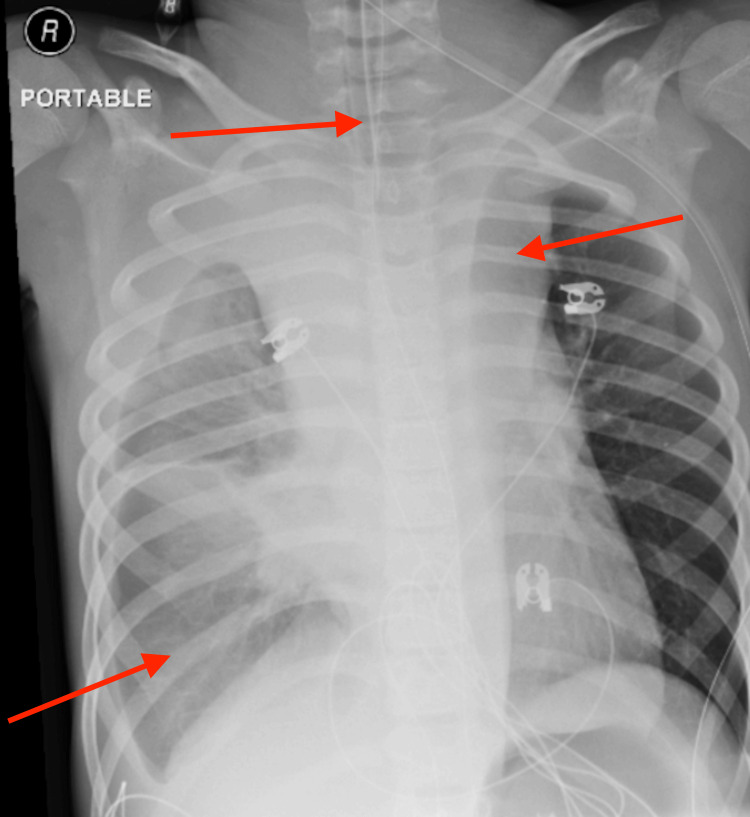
Portable supine anteroposterior chest radiograph The image demonstrates a widened mediastinal silhouette, right-sided pleural effusion, and associated right lung atelectasis (red arrows). These findings are consistent with a space-occupying mediastinal process with suspected mass effect on the central airway.
